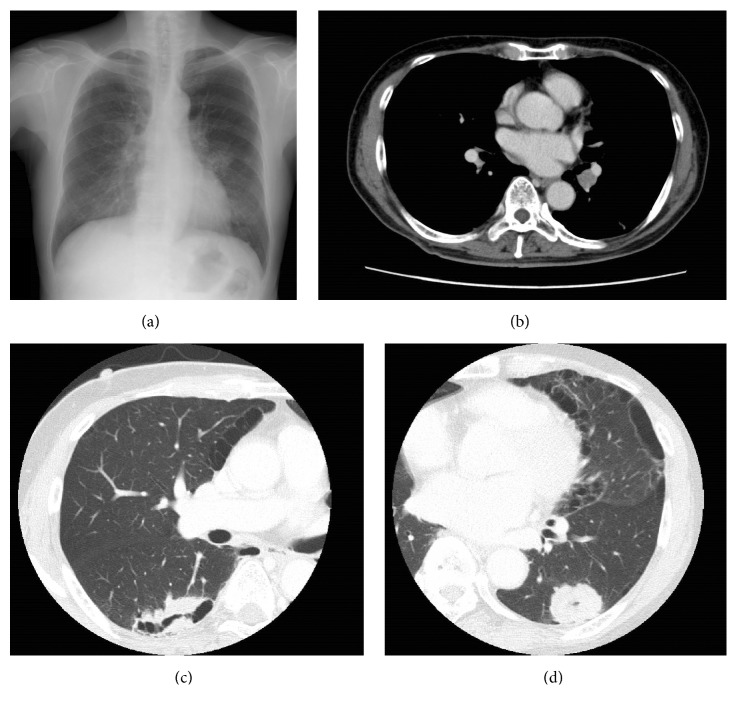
Radiologic examinations at the first visit to our hospital. (a) Chest X-ray. (b–d) Contrast-enhanced CT scan. (b) The left hilar lymph node. (c) The mass in the right lung. (d) The mass in the left lung.
